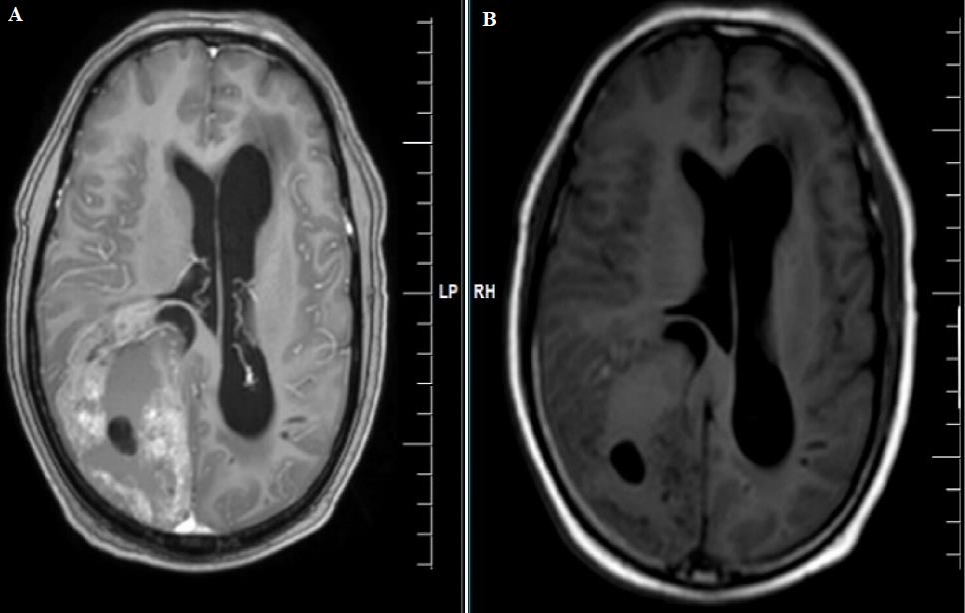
A,B) MRI of the brain axial section showing post-contrast enhancement in right occipital lobe
